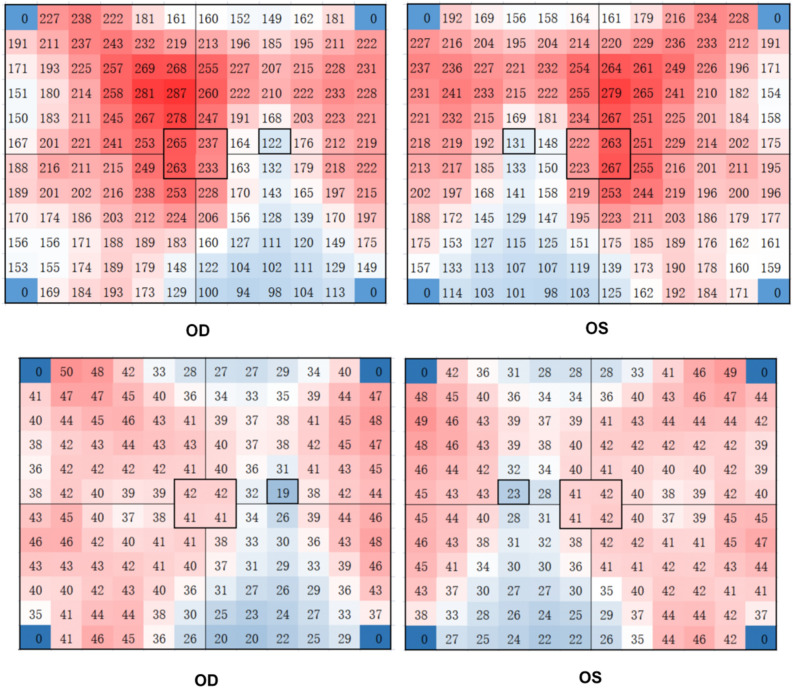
Topography of choroidal grid thickness (top) and topography of density of medium and large vascular layer (Sattler’s layer and Haller’s layer) in the choroid (bottom) for all participants. The darker the red, the thicker the CT in this area; the darker the blue, the thinner the CT in this area. The 4 mm × 3.3 mm area around the fovea was the four small squares surrounded by a black square in the middle, and the center of the macula was in the middle of the four small squares. The optic disc located at 4 mm from the nasal side of the fovea in a 2 mm × 1.7 mm area. Two vertical lines through the macular area divided the other areas into four parts: the supratemporal region, the supranasal region, the inferotemporal region, and the inferonasal region. All subjects followed this division method. This image was generated by Microsoft Excel in version 2021(https://hilcodigital.com/excel/) .
